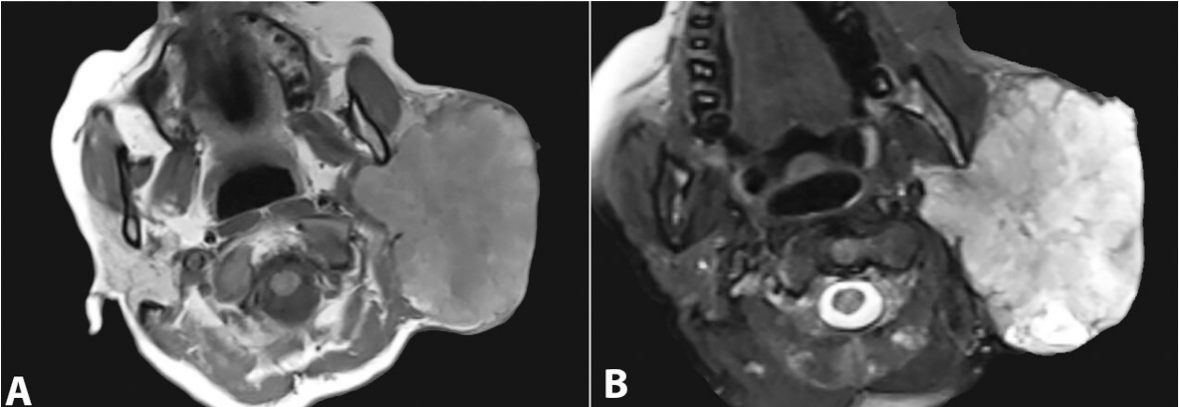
Head and Neck MRI . A – T1 weighed image showing a of lobulated parotid mass with hypointense signal; B – the mass shows hyperintense signal in T2 weighed image.
